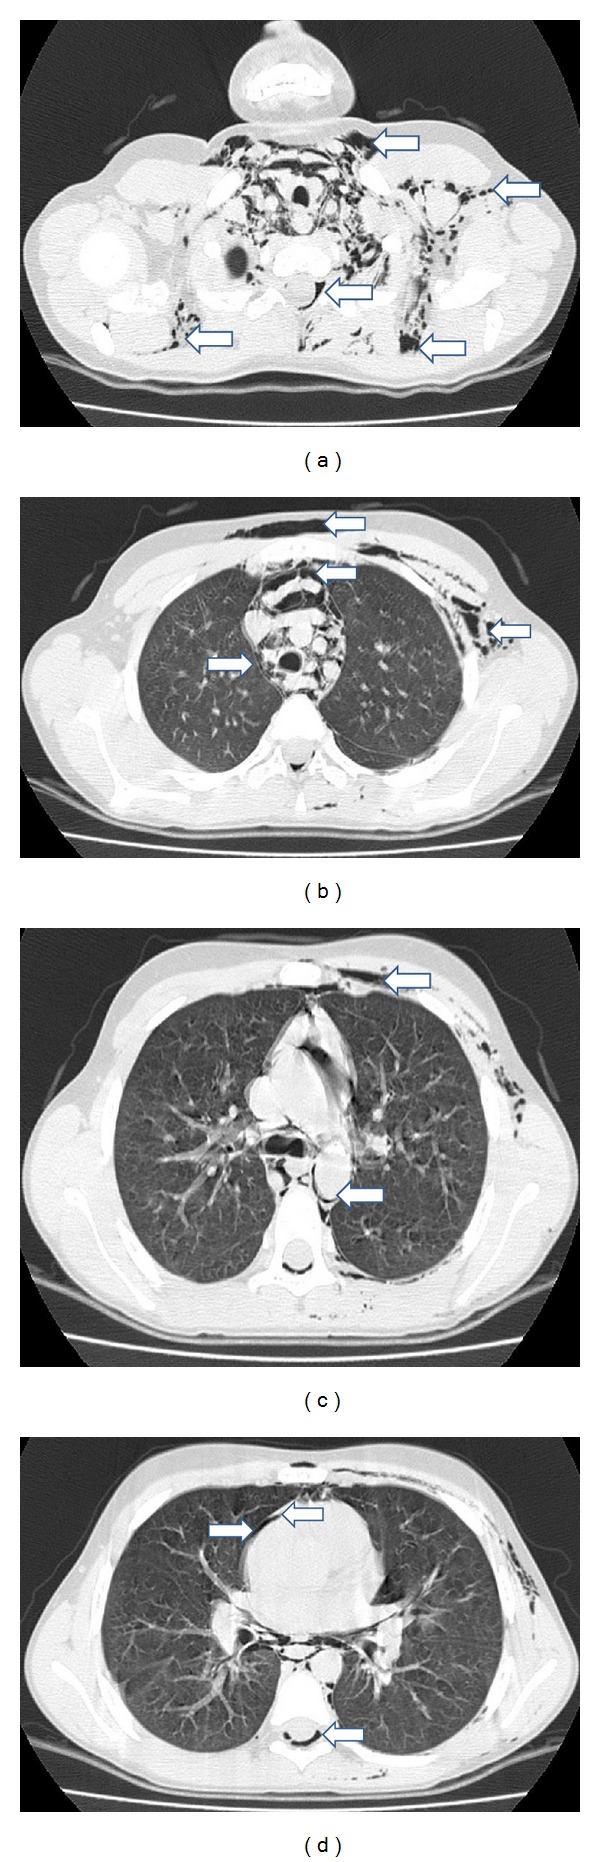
Chest computed tomography of the patient. (a) Free air in the soft tissues of the neck, subcutaneous emphysema, and air in epidural space of cervical vertebra. (b) Subcutaneous emphysema in the soft tissues of the left hemithorax and anterior chest wall as well as mediastinal emphysema surrounding mediastinal structures. (c) Free air surrounding mediastinal vascular structure and subcutaneous emphysema. (d) Pneumopericardium and air in the epidural space of the thoracic vertebra.
